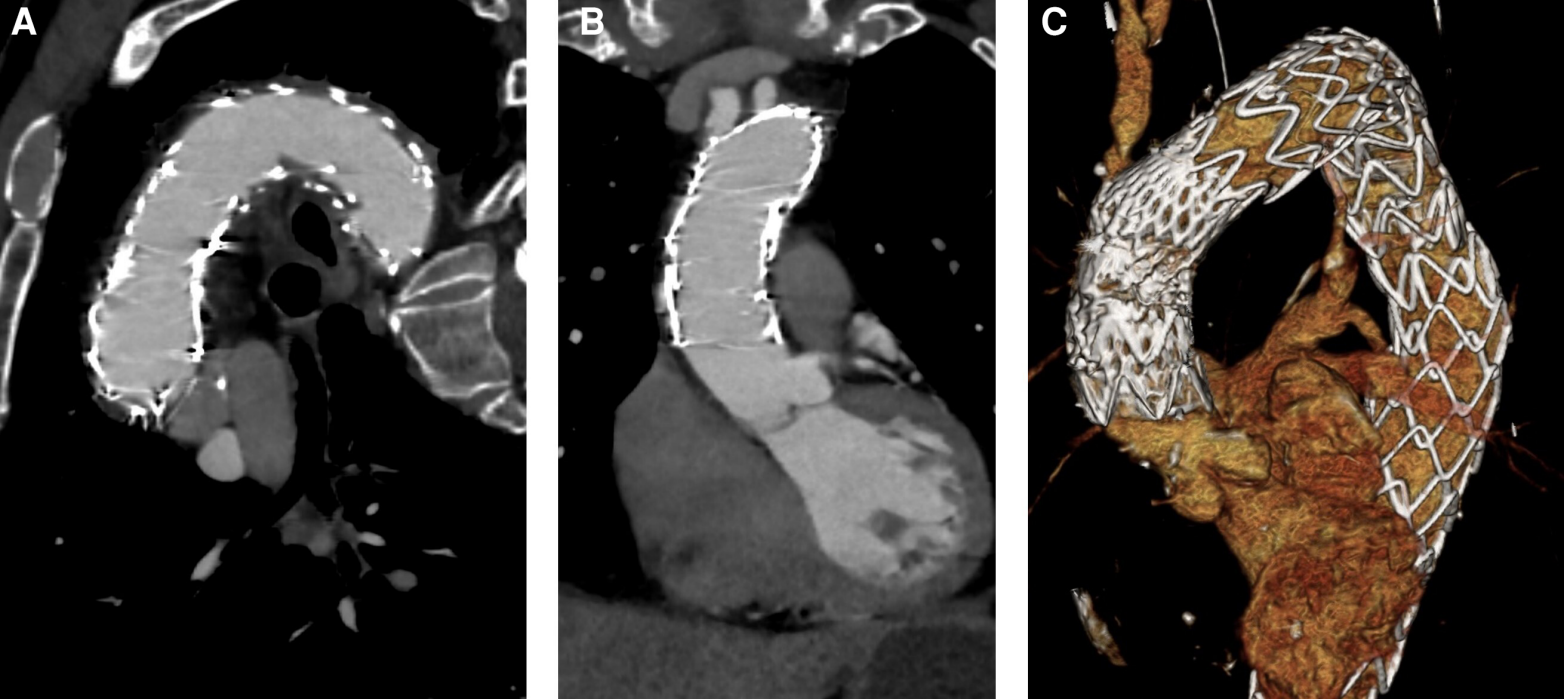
Postoperative CTA. (A) Stent grafts covering the ascending aorta and the aortic arch with no patent false lumen. (B) No residual dissection in the ascending aorta. (C) 3D reconstruction.
